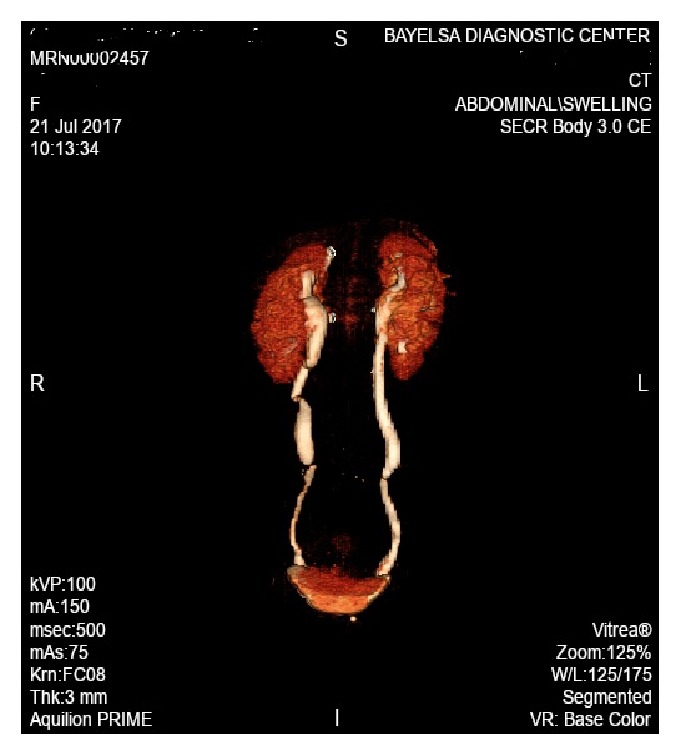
Presurgical evaluation outlining the kidneys, ureters, and bladder on contrast CT scan.
